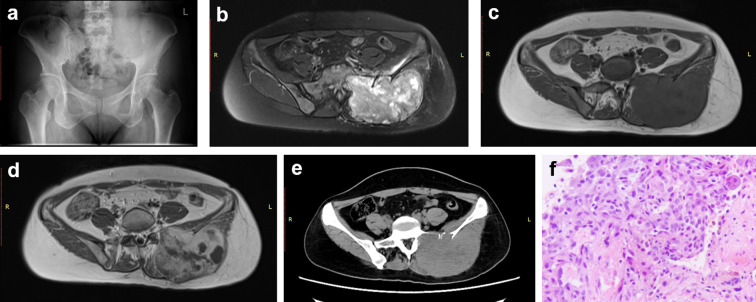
Case 3. A 47-year-old man was diagnosed with the giant cell tumor of bone. X-ray revealed an unclear sacroiliac joint space (a). MRI showed heterogenous high intensity on T2-weighted (fat suppression) images (b) and low intensity in T1-weighted images (c). The T1-weighted imaging also displayed a mixed enhancement pattern (d). No calcification was observed on the CT scan (e). Neither the actual nor the predicted pathological diagnoses (f) were pelvic chondrosarcoma.
